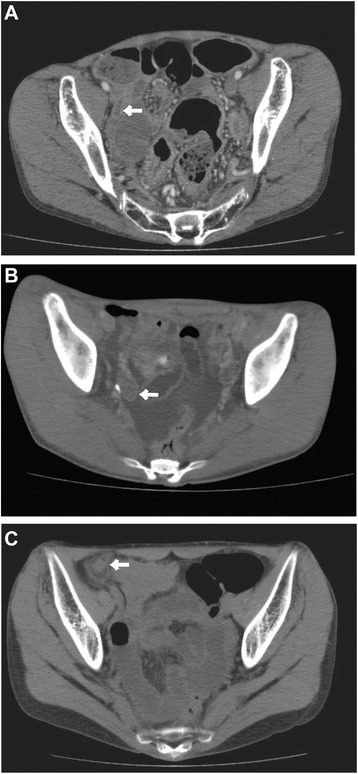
Images of abdominal CT. a Case 1, b Case 2, c Case 3. *Arrow* indicates swollen appendix
